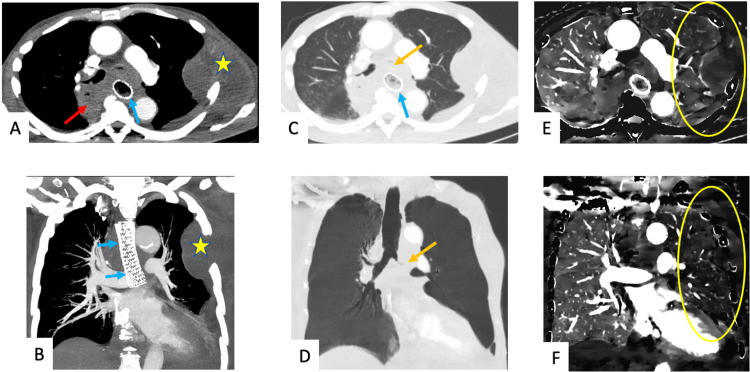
A 66-year-old male with advanced unresectable esophageal adenocarcinoma, status post stenting, presenting with shortness of breath. Axial and coronal CT images of the chest in soft tissue (A, B) and lung window (C, D) settings demonstrate a mass within the posterior mediastinum (red arrow), correlating with the patient's known primary esophageal malignancy with a stent in place (blue arrows) and associated complete obliteration/invasion of left principal bronchus (tan arrows). A left lateral chest wall metastatic mass is also visualized (yellow stars). On corresponding axial and coronal iodine density images (E, F), there is diffusely and significantly decreased perfusion throughout the left lung (yellow oval), likely secondary to hypoxic vasoconstriction from more proximal left mainstem bronchial occlusion. CT: Computed tomography
